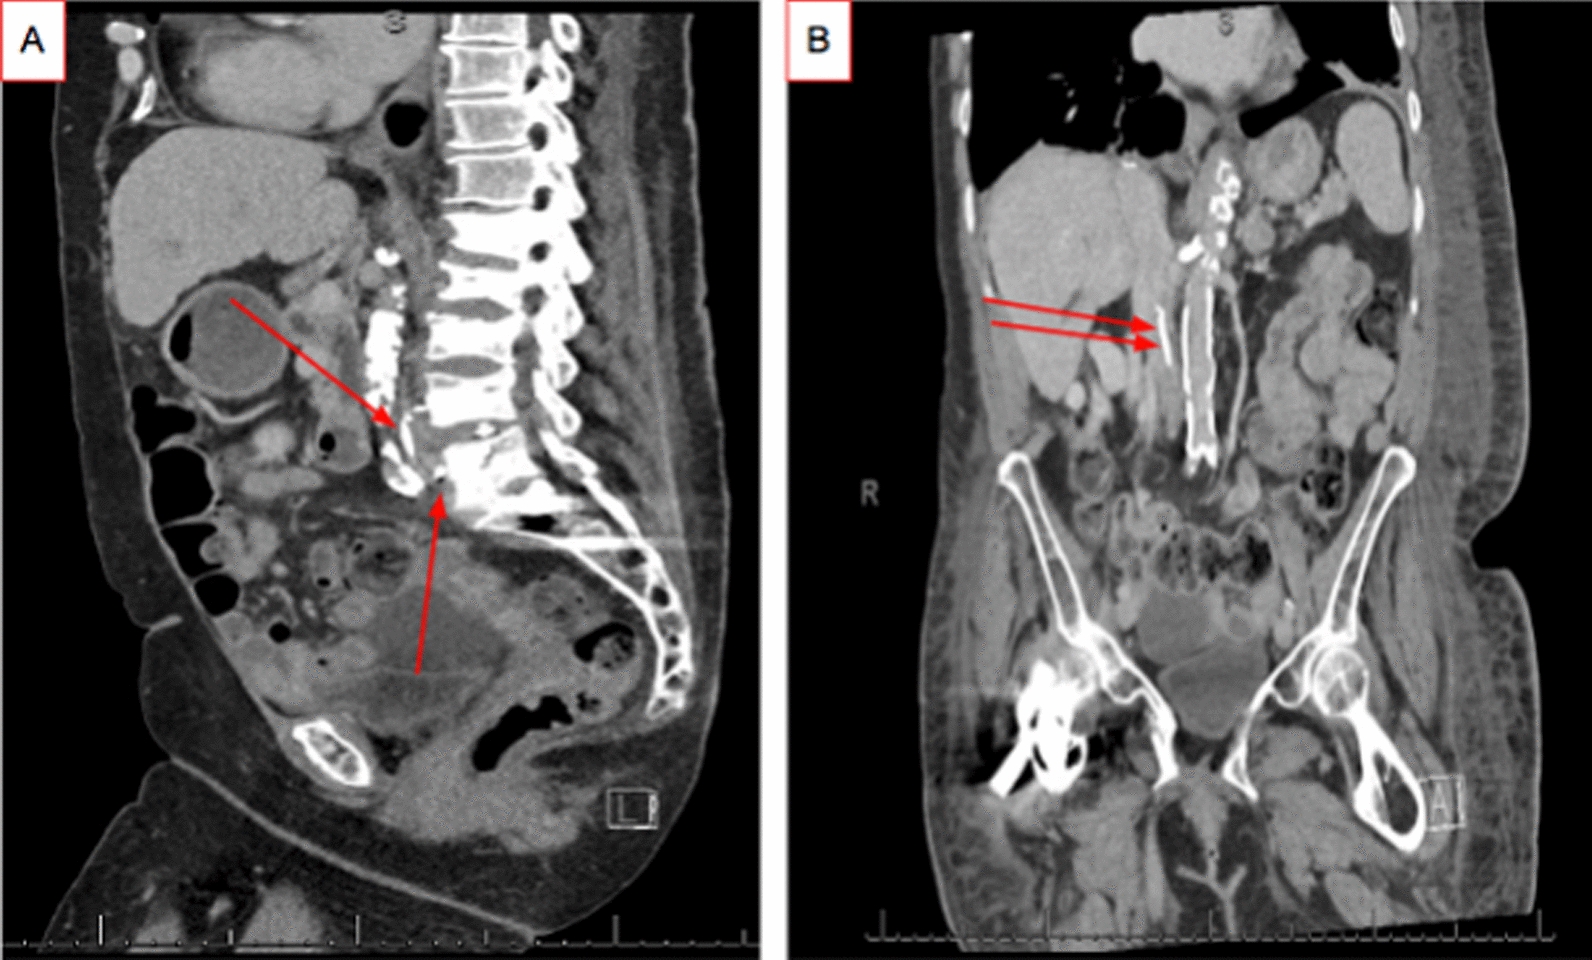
CT abdomen/pelvis, including sagittal (A) and coronal (B) views with red arrows showing cement extravasation in relation to calcified aorta
